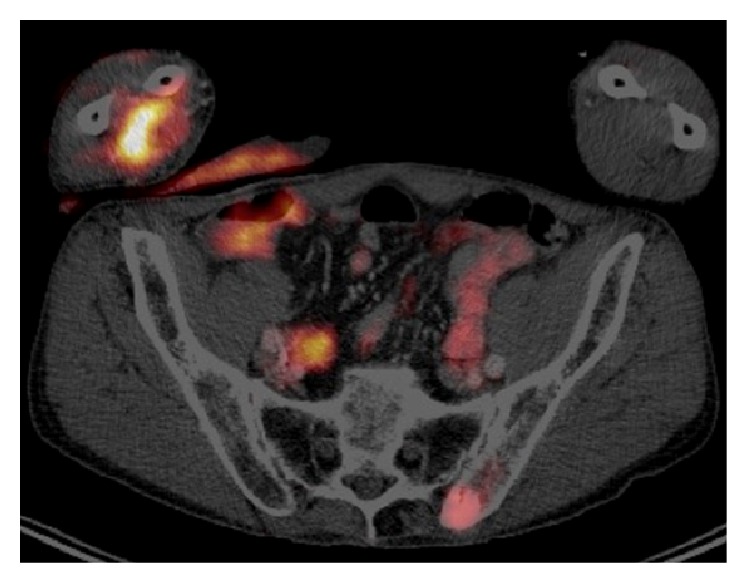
A Pet/CT whole body showed an advanced stage of the cancer, with multiple metastasis in the right forearm and in the left sacroiliac joint and the chest wall.
